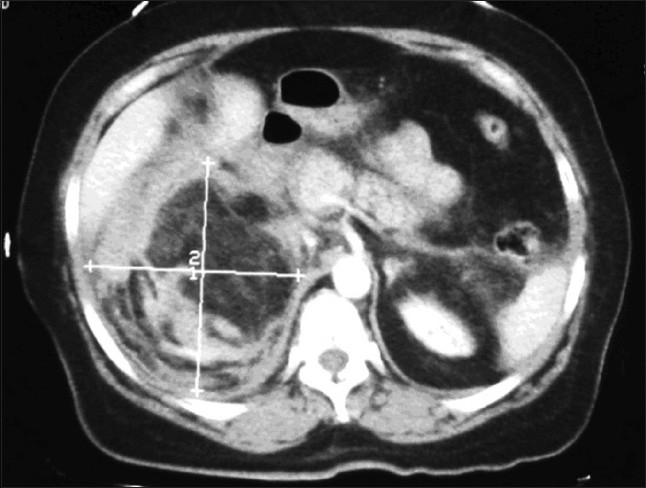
Abdominal computed tomography scan showing a 22 × 15 cm right renal fat mass with a perirenal haematoma in the right kidney
